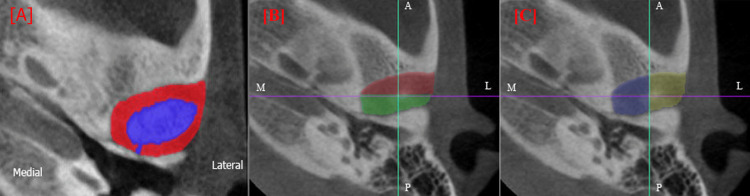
Three-dimensional volumetric measurement of the TMJ space from the axial plane in CBCT (A) Volume of the glenoid fossa (combined red and blue) and the TMJ space (only red area); (B) Division of the TMJ space in the anteroposterior direction; (C) Division of the TMJ space in the mediolateral direction A: anterior; P: posterior; M: medial; L: lateral; TMJ: temporomandibular joint; CBCT: cone-beam computed tomography
